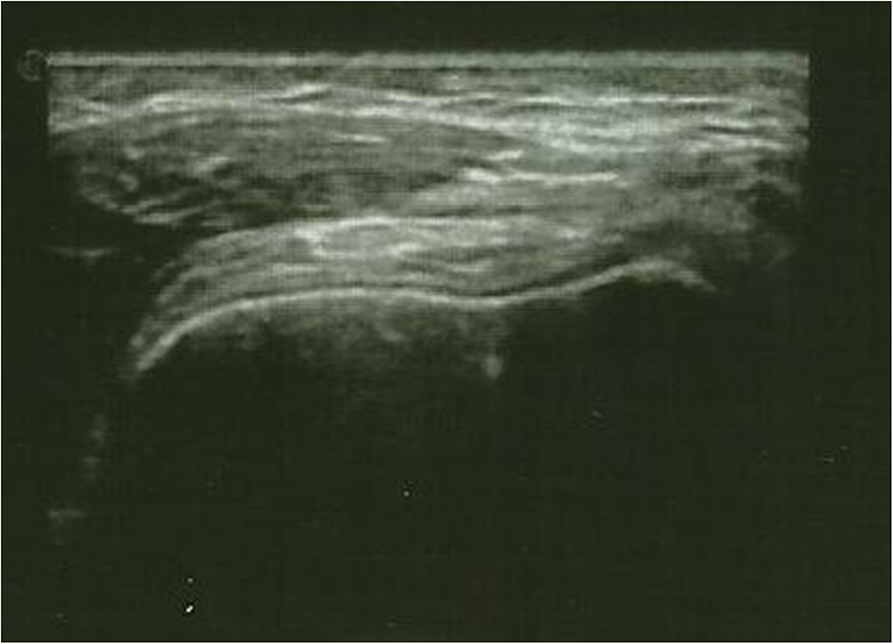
Case 1 left knee ultrasound after treatment. Left knee: transverse gray-scale 12–5 MHz ultrasound image obtained over the medial aspect of the knee showing normal aspect of the joint without fluid or synovial pannus into the subquadriceps recess.
